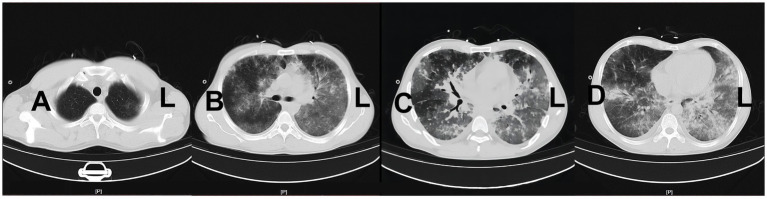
Reexamination of chest CT on the 15th day of admission before the application of ECMO.
